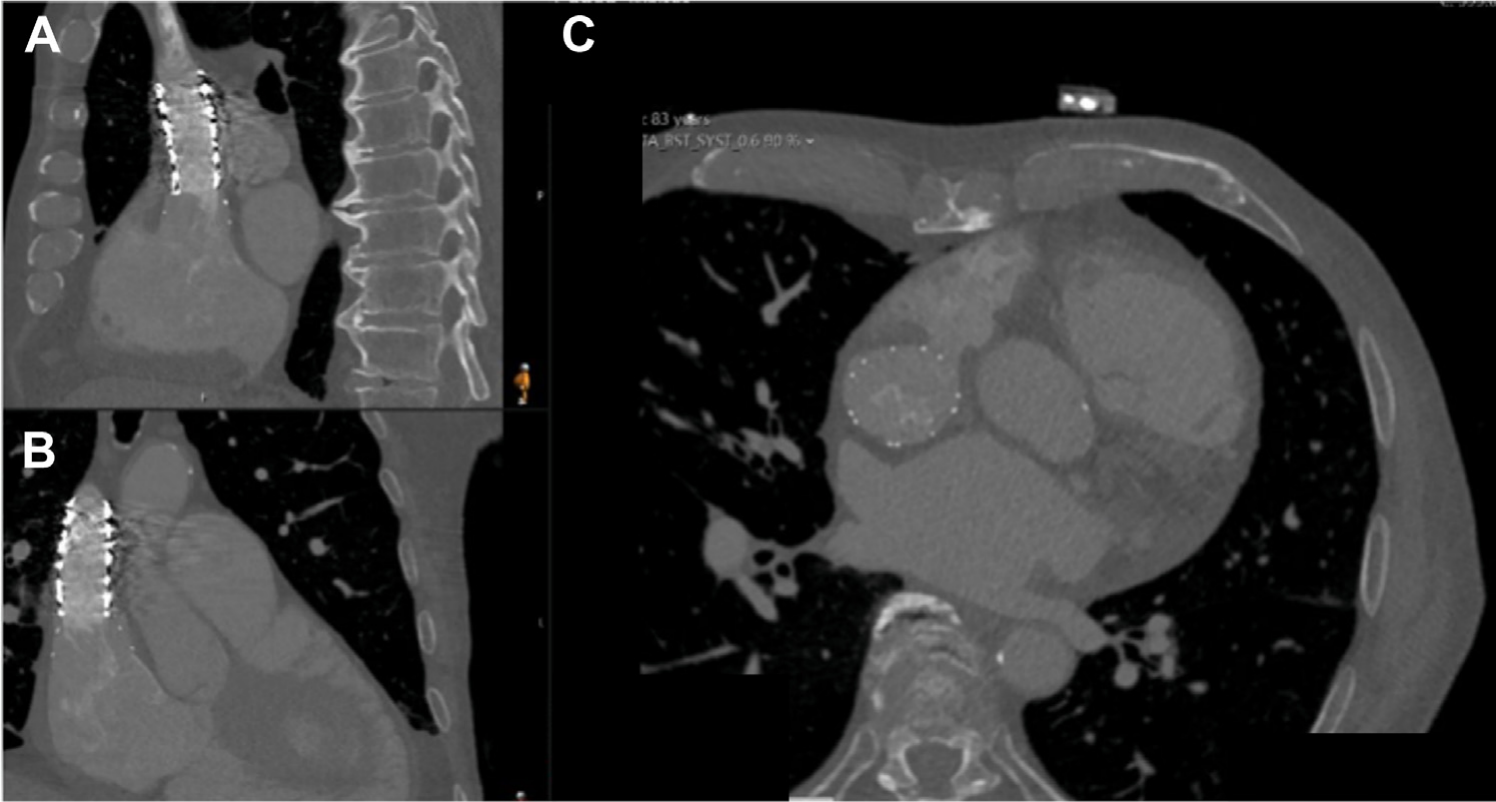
Follow-Up Multiplanar Computed Tomography Imaging Follow up (A) sagittal, (B) coronal, and (C) axial computed tomography images showing a stable Harmony Transcatheter Pulmonary Valve 22 mm (Medtronic) and covered stent placement, with complete occlusion of the sinus venosus atrial septal defect.
